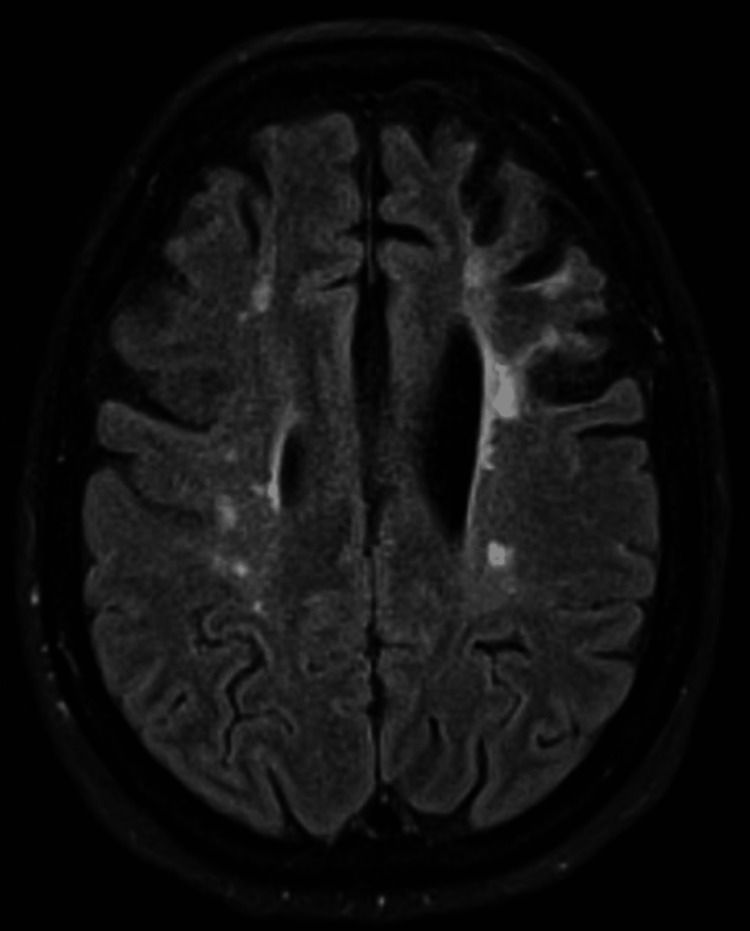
FLAIR-weighted MRI showing a corticosubcortical frontal hyper-intensity with, mostly, frontal cerebral atrophy FLAIR, fluid-attenuated inversion recovery; MRI, magnetic resonance imaging
